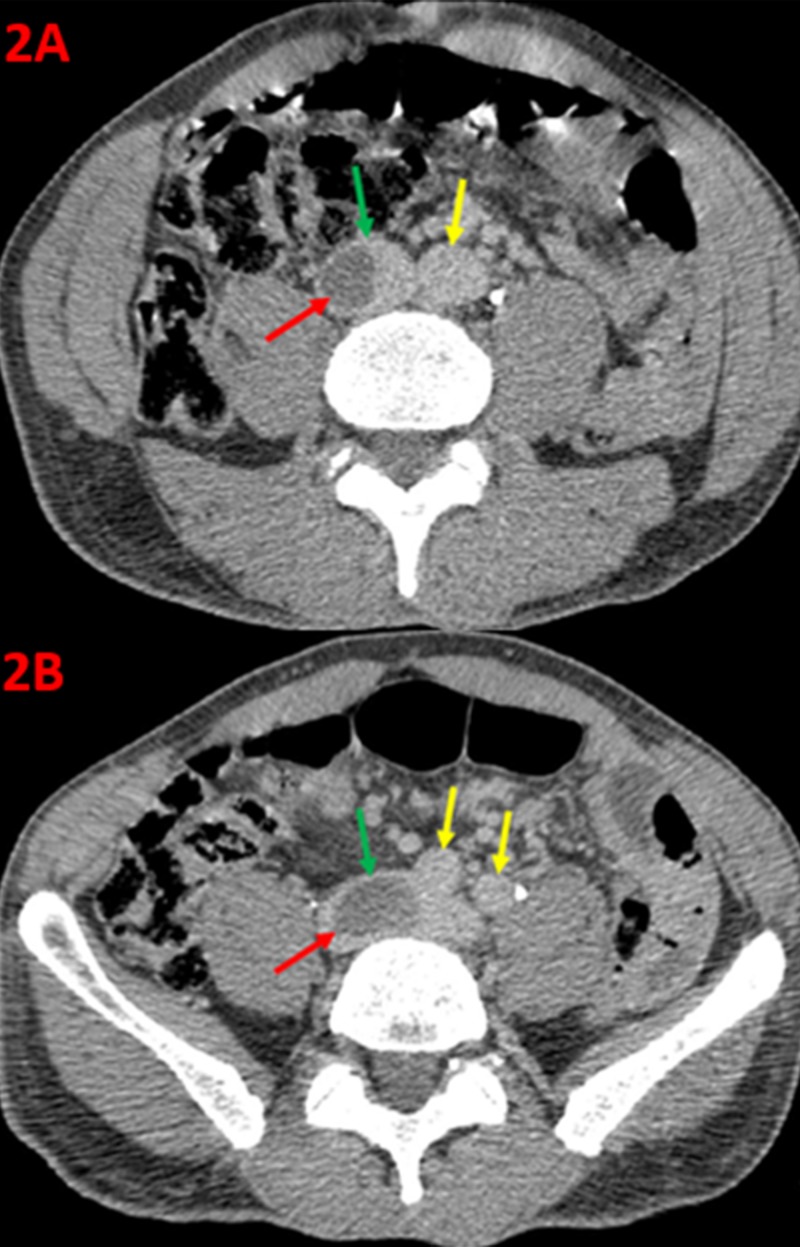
Axial Computed Tomography of the Abdomen and Pelvis An axial contrast-enhanced computed tomography of the lower abdomen (2A) demonstrates the aorta (yellow arrow), the inferior vena cava (green arrow), and a filling defect (red arrow) within the inferior vena cava, consistent with a thrombus just superior to the confluence of the iliac veins. An axial contrast-enhanced computed tomography of the pelvis (2B) demonstrates the right and left common iliac arteries (yellow arrows), the inferior vena cava (green arrow), and a filling defect (red arrow), within the confluence of the inferior vena cava with extension into the proximal right common iliac vein.
